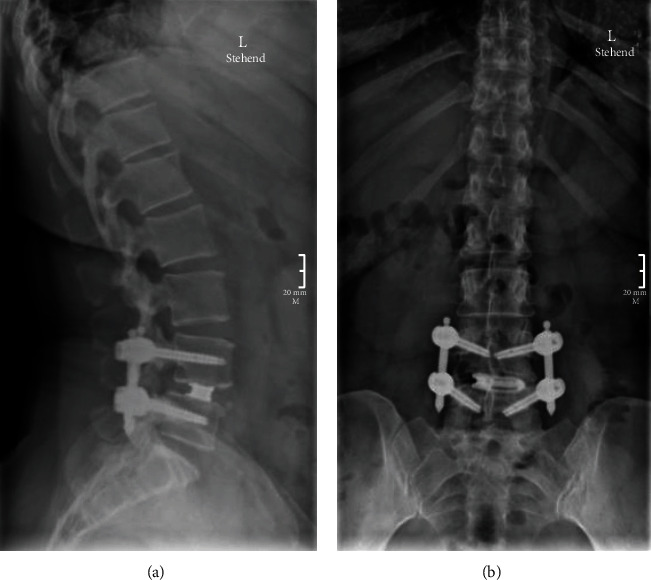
Routine follow-up X-ray status postendoscopic TLIF. (a) Postoperative lateral films demonstrate reduction in L4-5 anterolisthesis with adequate cage placement. (b) Normal percutaneous pedicle screws and interbody cage without evidence of hardware complication.
